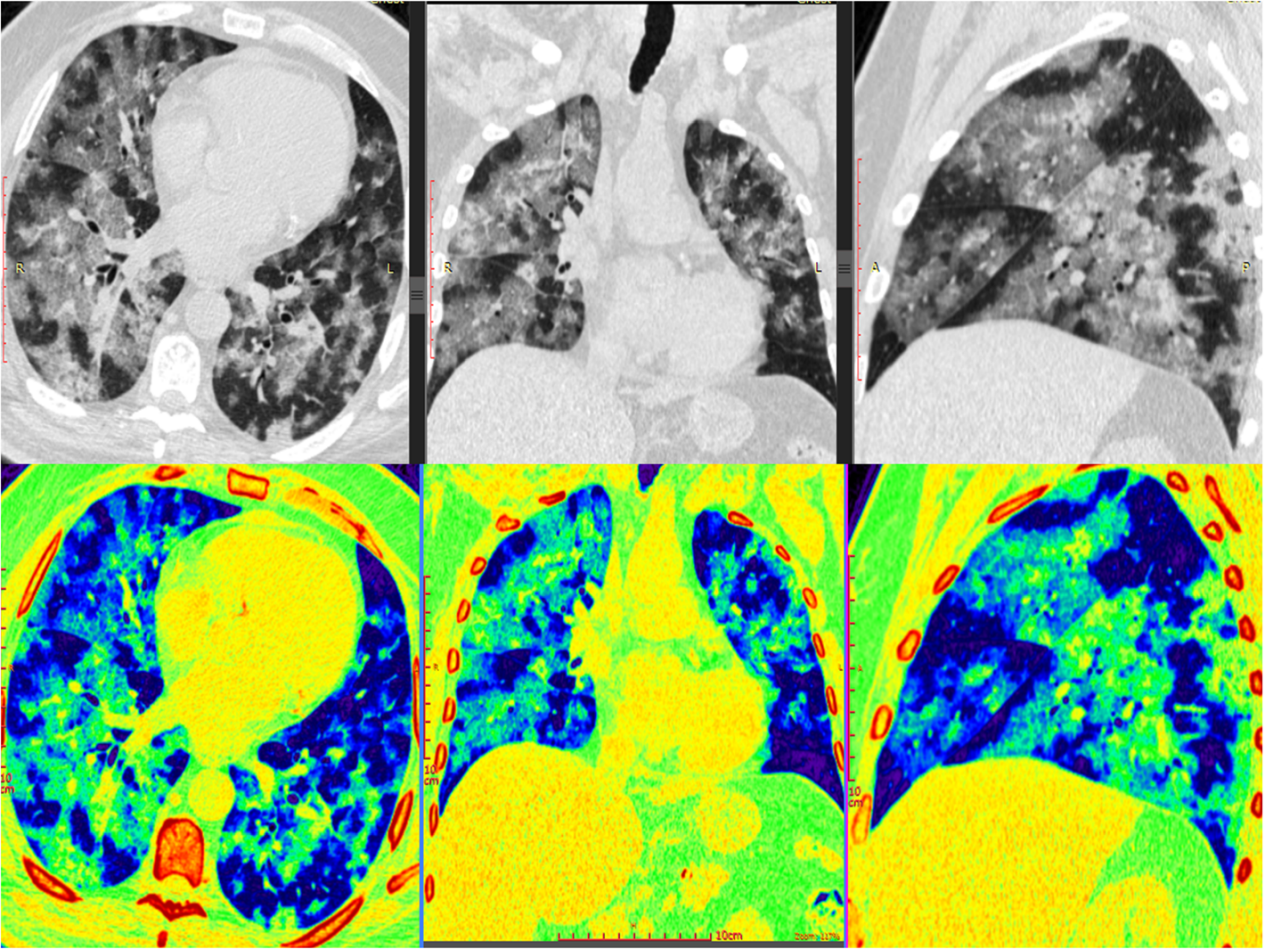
A 55-year-old female patient presented with fever, dyspnea, and cough. MSCT scan with multi-planar and color-coded images showed widespread confluent bilateral peripheral and central predominant ground glass opacities with septal and vascular thickening. CT-SSS was 19 and serum LDH was 550. The patient exhibited critical disease course with ICU admission, invasive mechanical ventilation, and finally died
